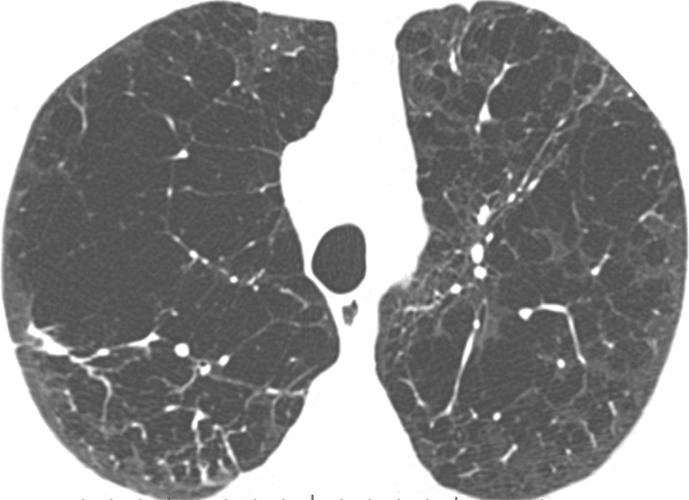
Axial CT images show severity grades of parenchymal emphysema. (a) Normal CT scan shows no emphysema. (b) Image shows trace centrilobular emphysema (circle), which involved less than 0.5% of the lung zone. (c) Image shows mild centrilobular emphysema (arrows), which involved 0.5%–5.0% of the lung zone. (d) Image shows moderate centrilobular emphysema, which involved more than 5% of the lung zone. (e) Confluent emphysema. (f) Advanced destructive emphysema with vascular distortion.
